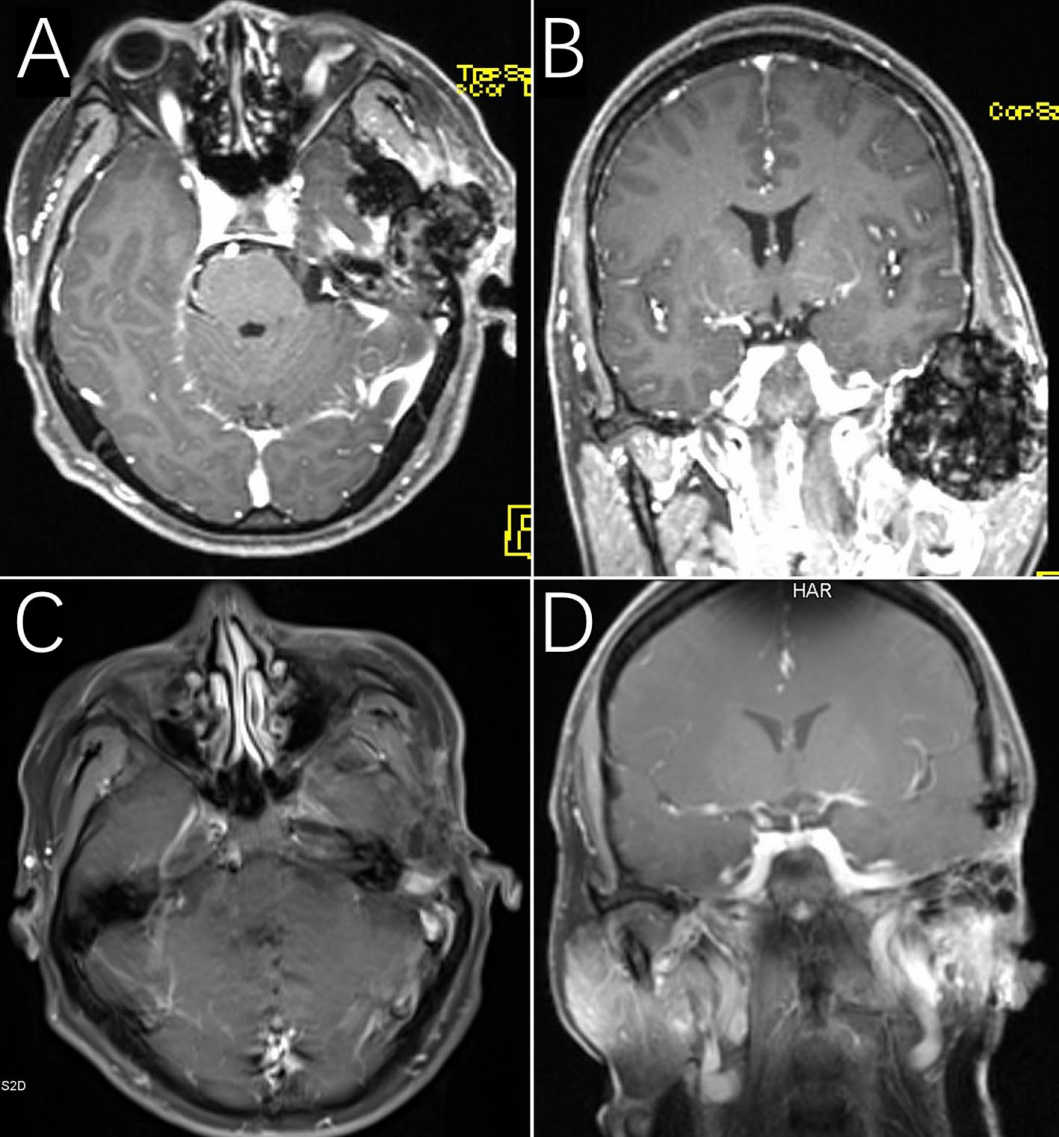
Patient 9: PVNS on MRI showed modest inhomogeneous contrast enhancement, expansive growth and skull base invasion (A and B). After 8 months of follow-up, the tumor had not recurred (C and D).
